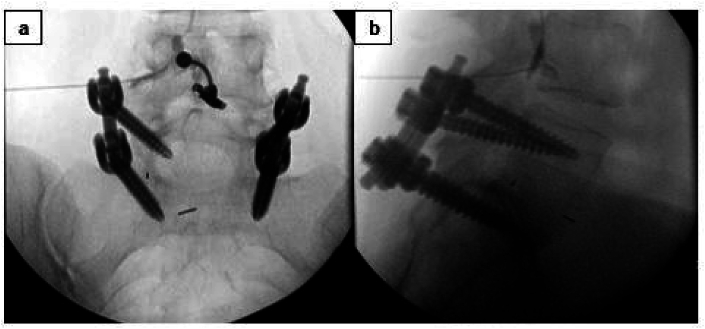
a) AP fluoroscopic radiograph depicting the epidural flow of contrast in TFESI prior to administration of injectate in same fashion b) Lateral view of same procedure depicting flow of contrast in the anterior epidural space.
